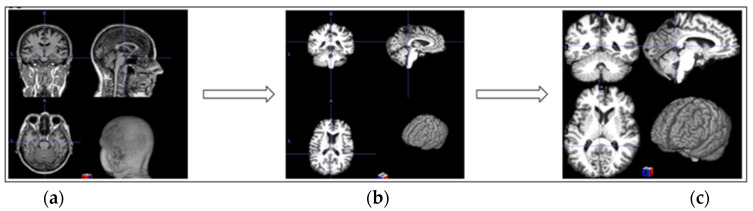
Three-dimensional MRI preprocessing: (a) original image; (b) preprocessed with FreeSurfer autorecon1; (c) background removal.
